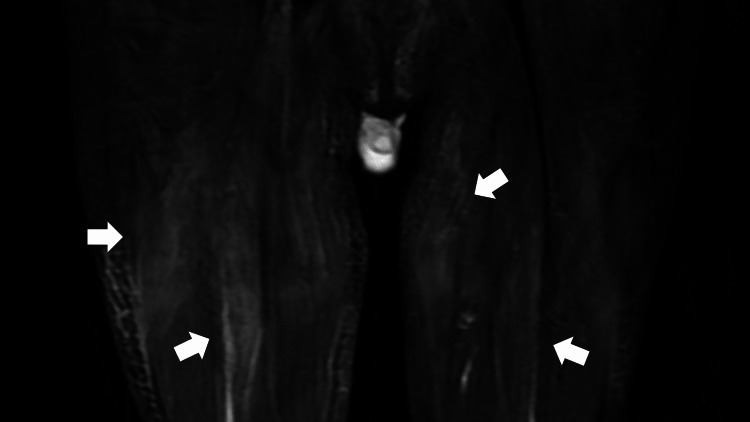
A femoral magnetic resonance imaging scan (coronal plane) showing the bilateral hip and thigh muscles with a high signal (arrows).
